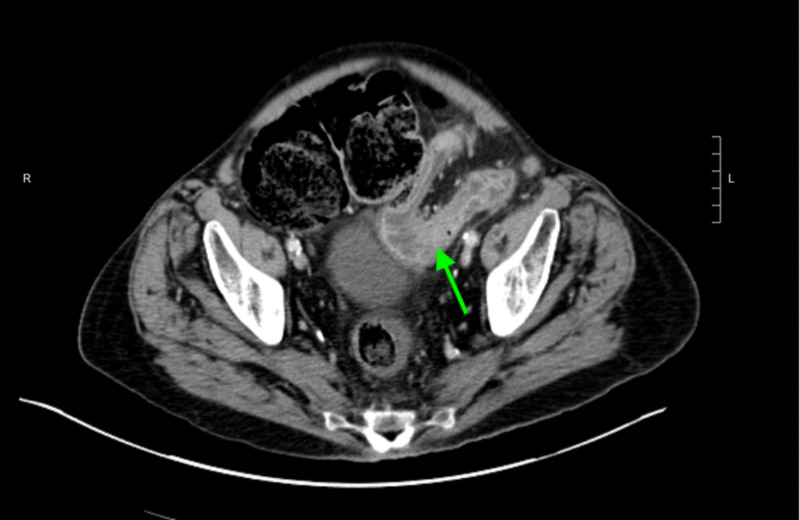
CT of the abdomen and pelvis with intravenous contrast demonstrating segmental inflammatory change involving the distal descending colon (arrow) suggestive of colitis.
